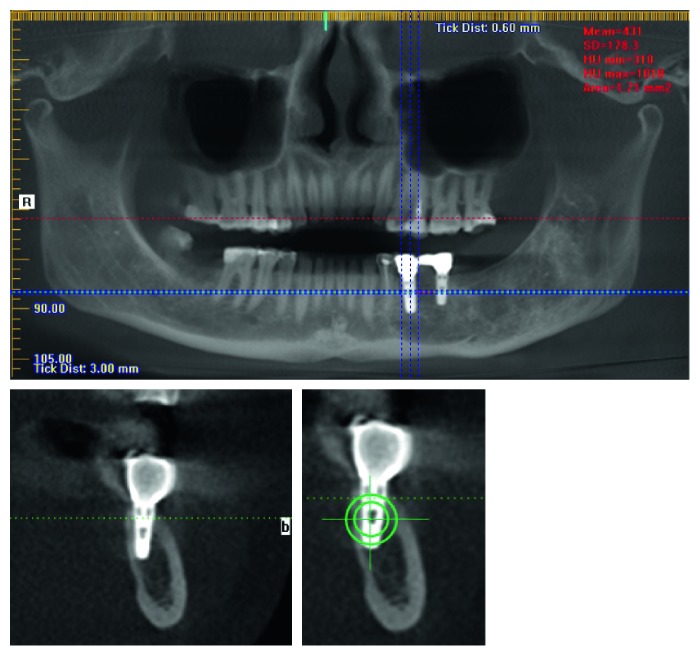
3D CT scans of dental implants. Measuring points for densitometry (green).
